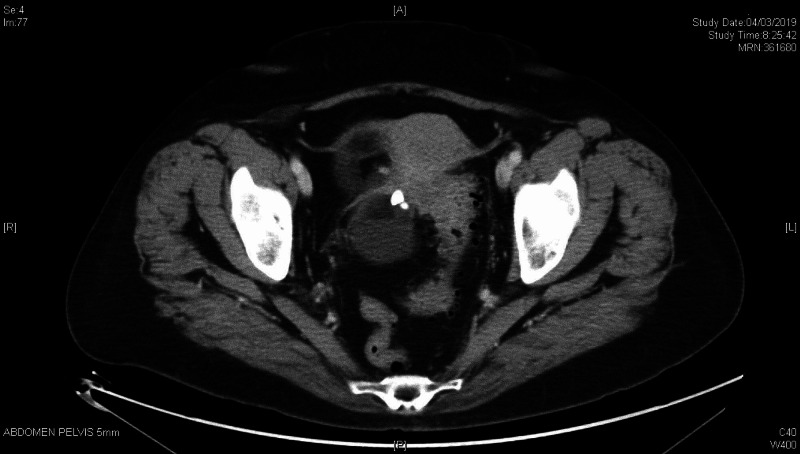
CT scan Right adnexal lesión; size 7.9x10.4x11.9 cm, presence of fat and calcium content inside, without solid pole images, suggestive of teratoma
